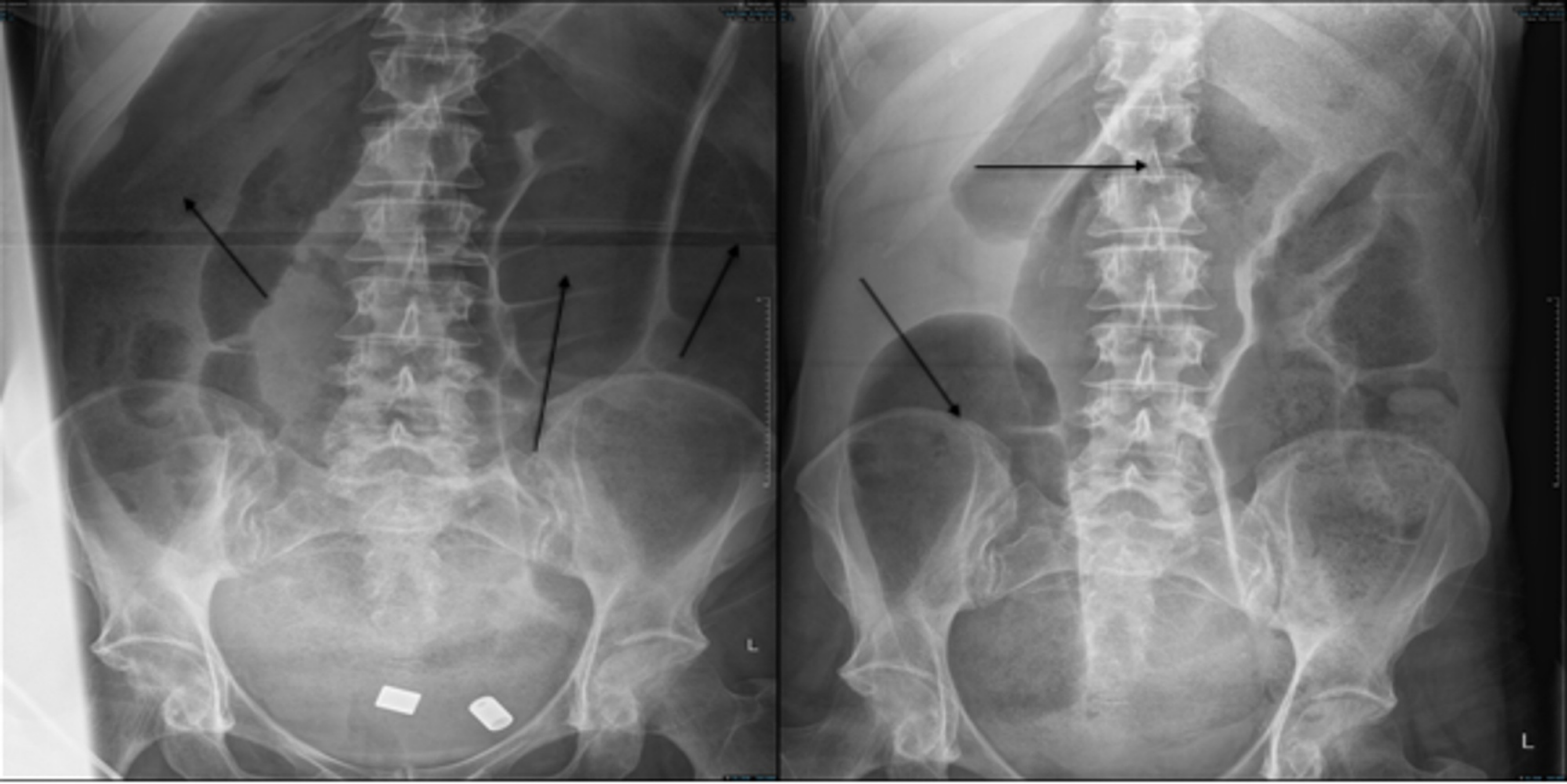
Plain abdominal X-ray showing dilated featureless loops of large bowel secondary to sigmoid volvulus
